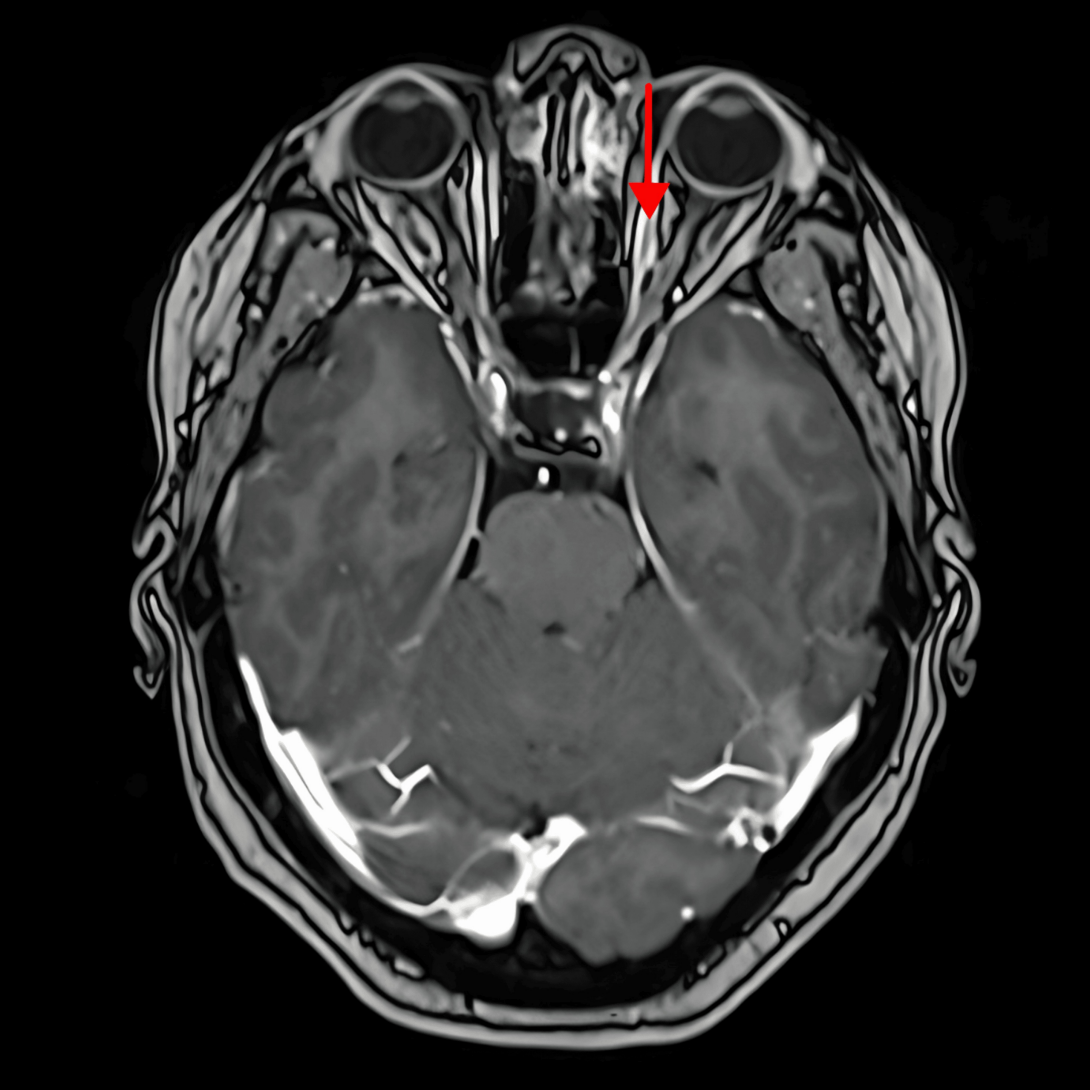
Brain MRI for follow-up post-radiotherapy: reduction in the size of the left intraorbital intracoronal lesion (7×4 mm) MRI: magnetic resonance imaging
